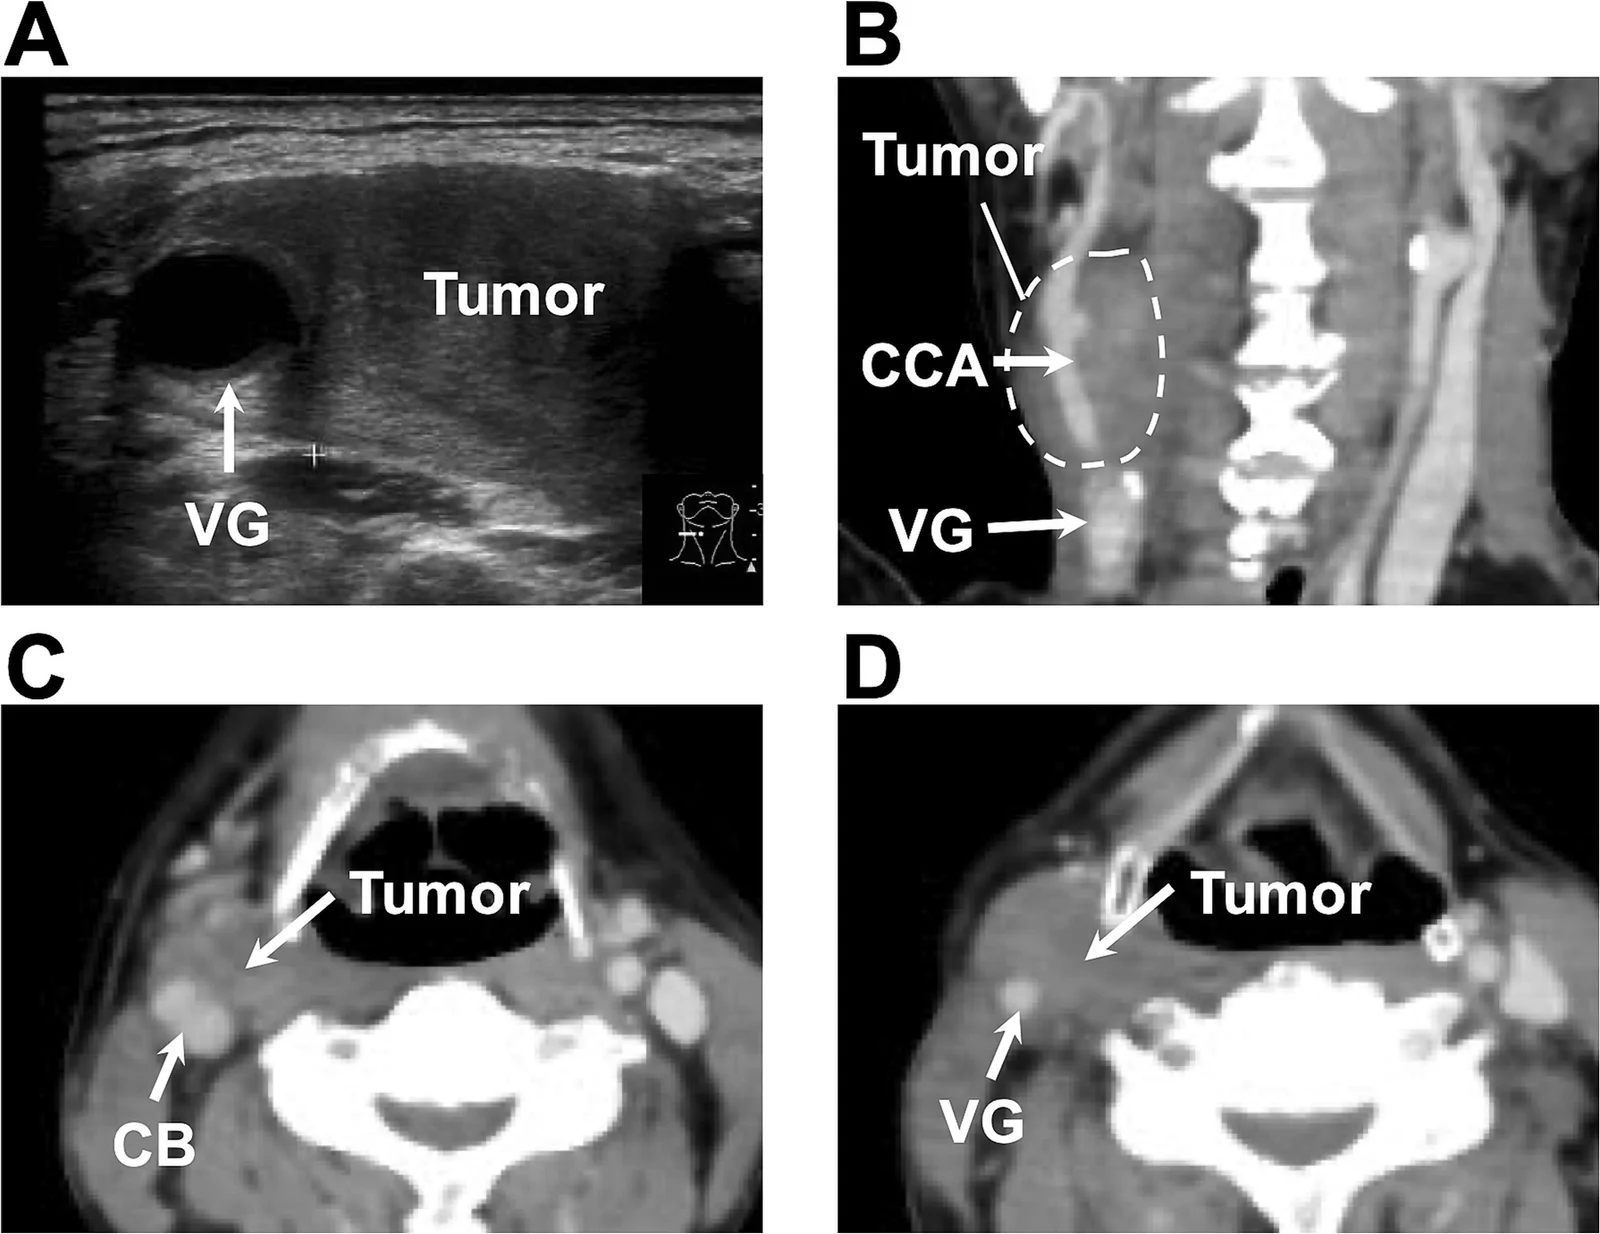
Clinical findings at the second recurrence. The tumor circumferentially surrounded the anastomosis between the common carotid artery and vascular graft. (A) Ultrasound examination. (B–D) Contrast-enhanced CT. CB, carotid bifurcation; CCA, common carotid artery; VG, vascular graft.
